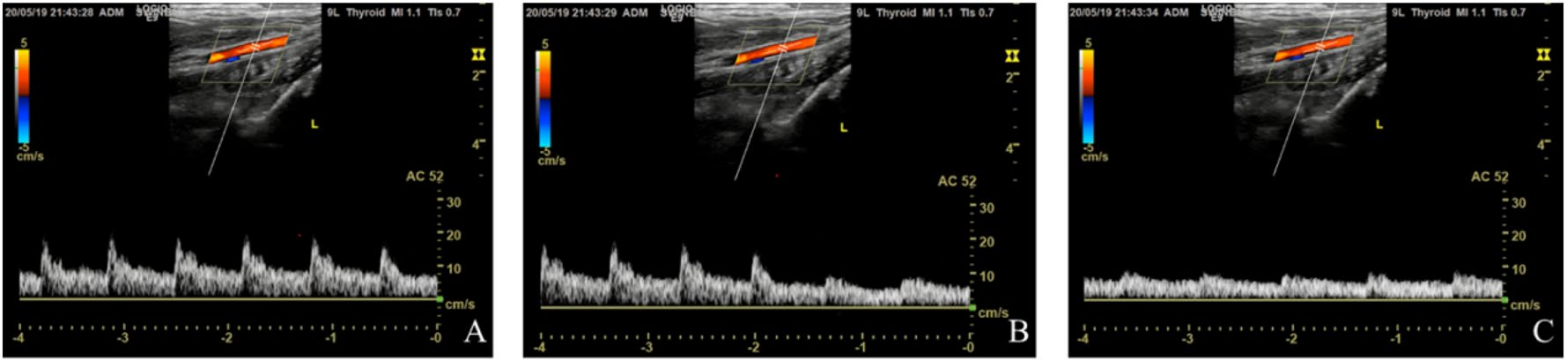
After the balloon inflated at the abdominal aorta, the right iliac artery was disappeared. A: It was the normal Doppler spectrum of the right iliac artery. B: With the balloon was inflating, the Doppler spectrum of the right iliac artery was changed. C: After the balloon inflated, the Doppler spectrum of the right iliac artery was disappeared
